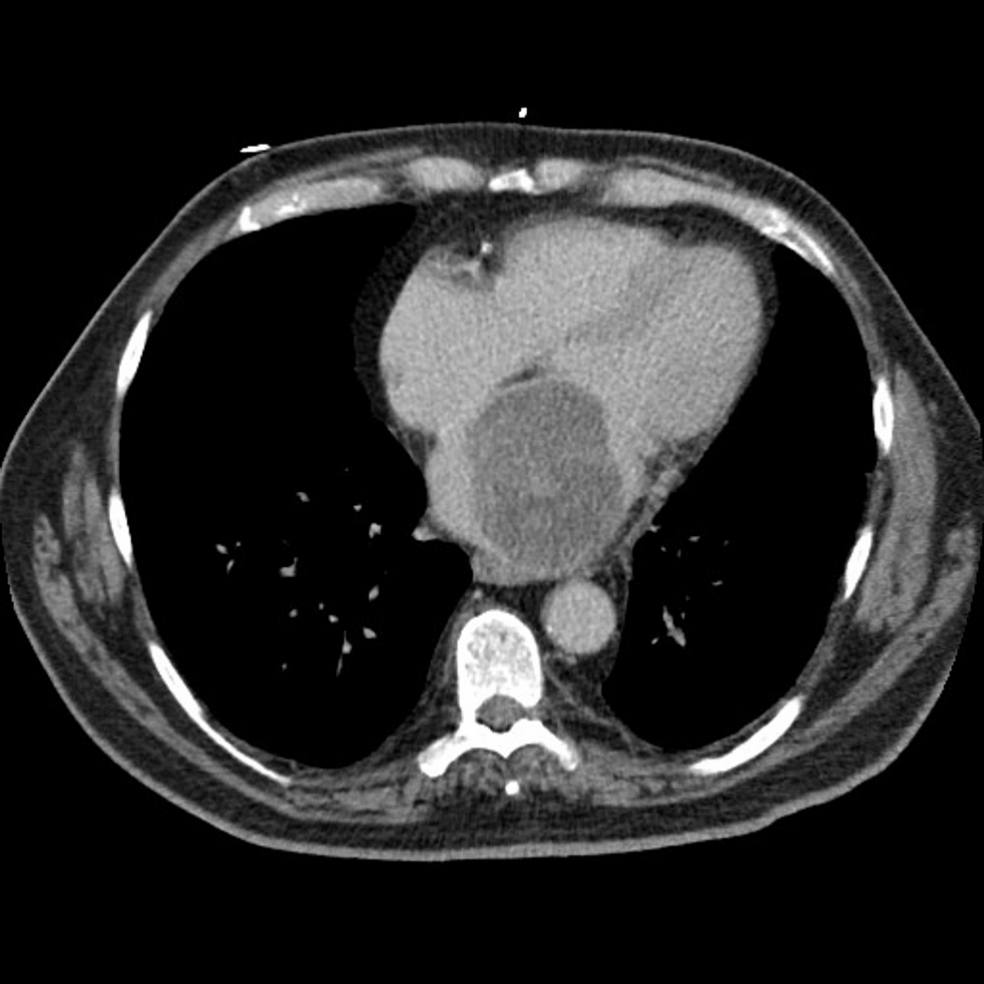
Left atrial filling defect noted on CT abdomen pelvis
